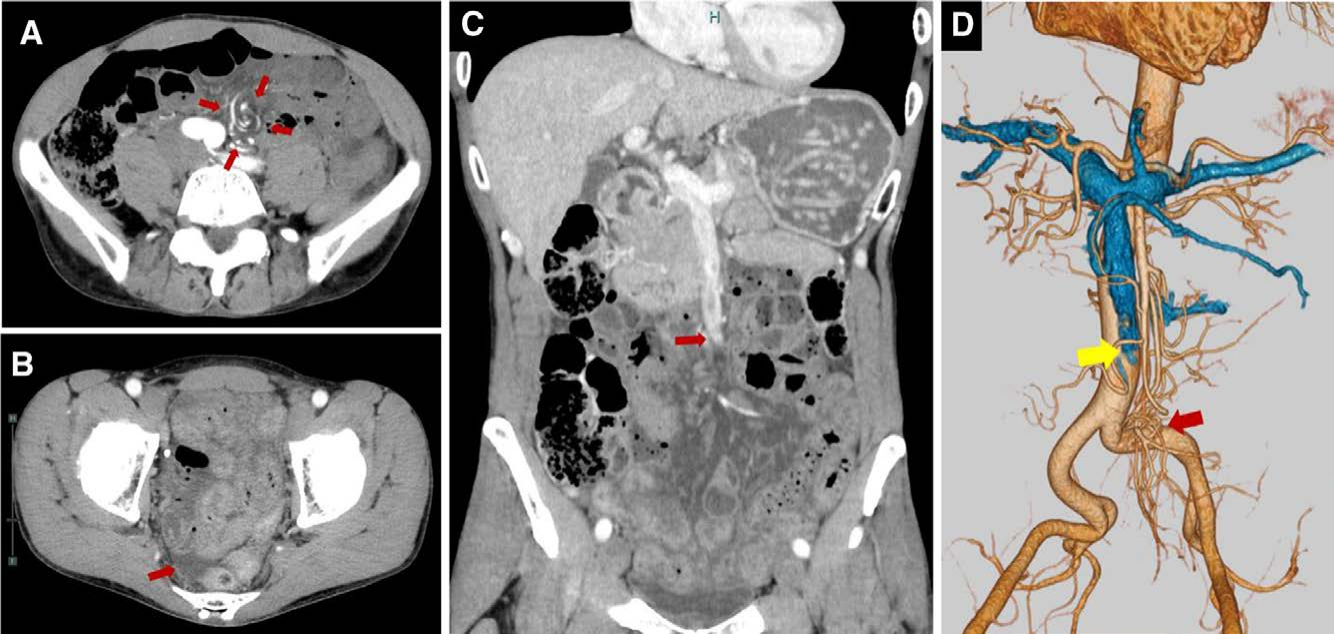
Preoperative contrast-enhanced computed tomography findings. (A) When the mesenteric base is viewed caudally, a 360°-clockwise rotation of the mesentery of the small intestine characterized by the rotation of branches of the superior mesenteric artery (SMA), known as the “whirl sign,” is revealed (red arrows). (B) Minimal ascites is present in the pelvic cavity (red arrow). (C) The rotation of the mesentery of the small intestine has resulted in the occlusion of the superior mesenteric vein (SMV; red arrow). Edematous changes in the mesentery of the small intestine, characterized by increased fat density, are visible. No obstruction or dilation of the small intestine is observed. (D) Three-dimensional angiography reveals small intestinal mesenteric torsion resulting in the occlusion of the SMV (yellow arrow) and rotation of the SMA with maintained blood flow (red arrow).
